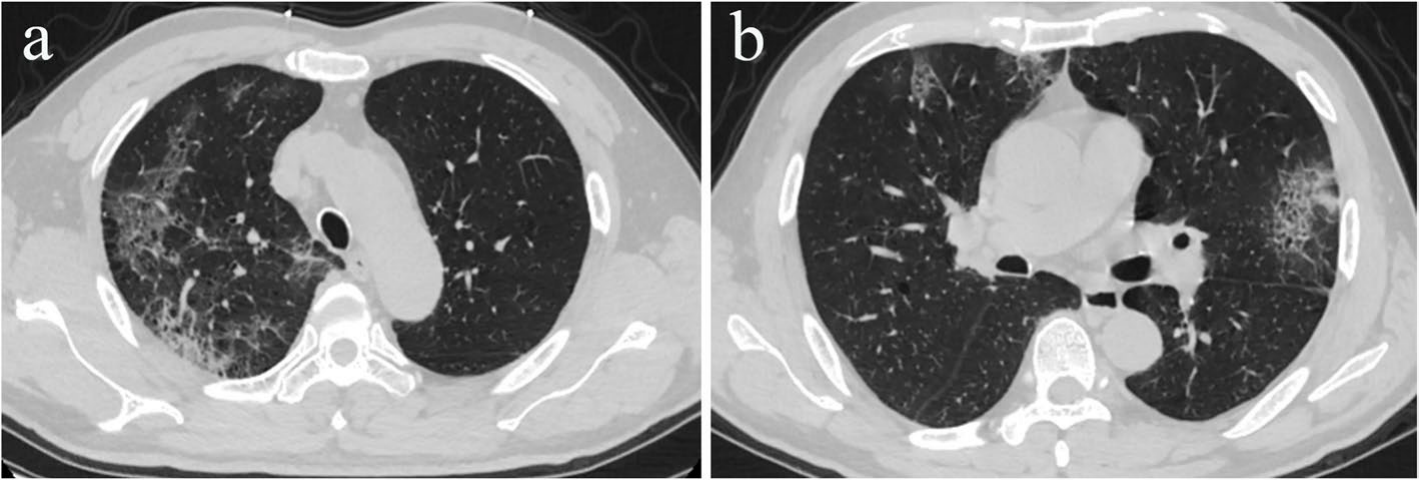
Chest CT shows ground glass-like shadows and multiple consolidations in the right upper lobe
